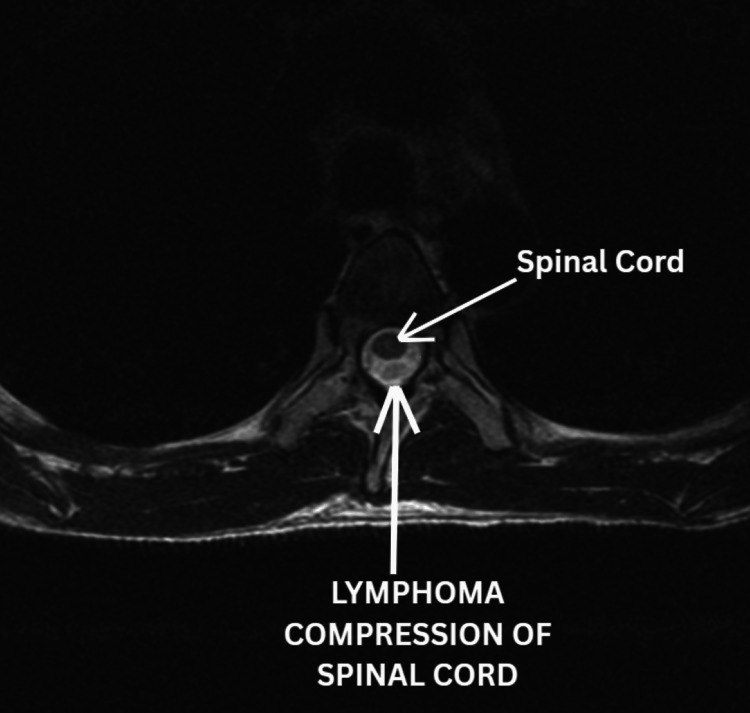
Axial T2-weighted MRI of the thoracic spine Axial T2-weighted MRI of the thoracic spine at the T2-T3 level demonstrates an expansile intramedullary lesion (arrow) with compression of the spinal cord by lymphoma. Abbreviation: MRI, magnetic resonance imaging.
